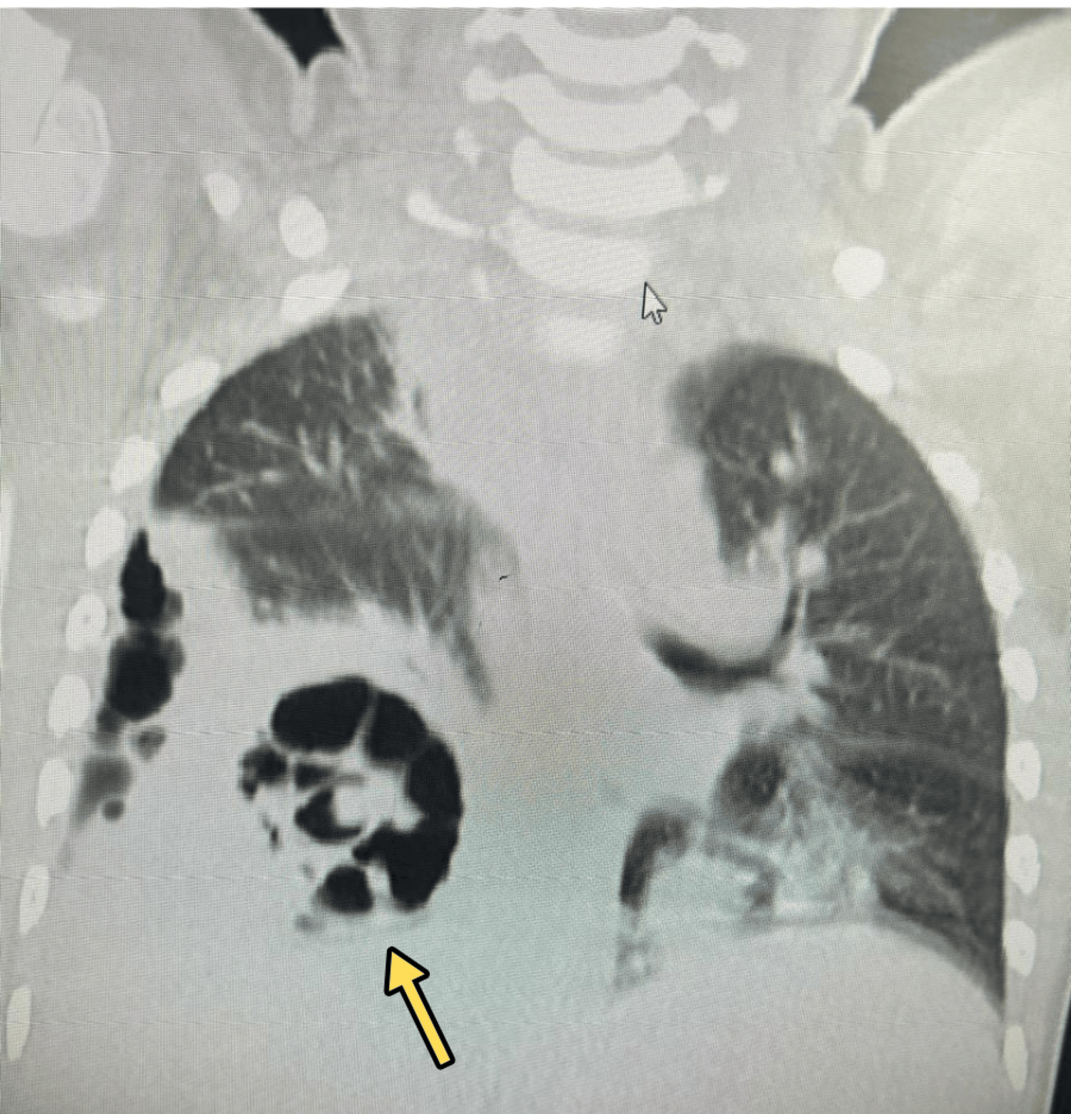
Contrast-enhanced computed tomography (CECT) of the chest showing necrotizing pneumonia Contrast-enhanced computed tomography of the chest with contrast showing dense consolidation in both lower lobes with cavitations (arrow), consistent with necrotizing pneumonia.
